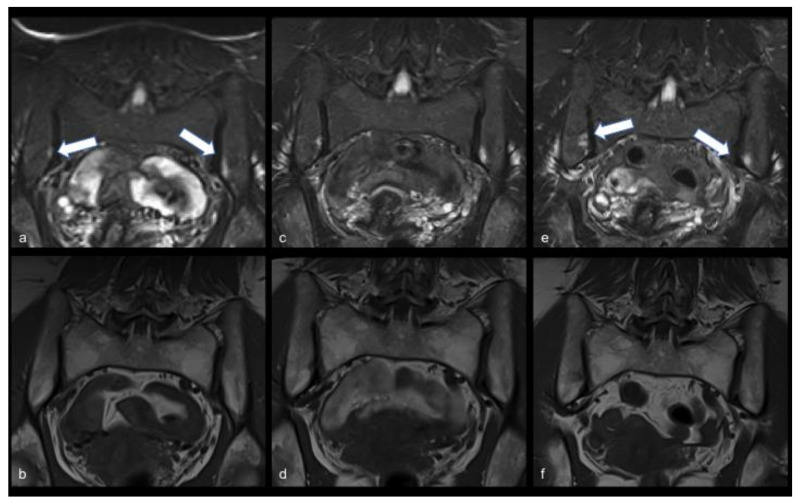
Case report 1. Persistent MRI findings suggestive of bilateral active sacroiliitis in a 58-year-old patient with lower back pain. On the first MRI examination, subchondral bone marrow oedema at the iliac side of both sacroiliac joints was found, appearing as band-like hyperintensity on short-tau-inversion recovery (STIR) T2-weighted semi-coronal image (arrows in (a)) with corresponding hypointensity on turbo-spin-echo (TSE) T1-weighted semi-coronal image (b). Oedema was found to persist at a second MRI examination performed one month later (c,d), and increase after six more months (e,f), especially on STIR T2-weighted image (arrows in (e)).
